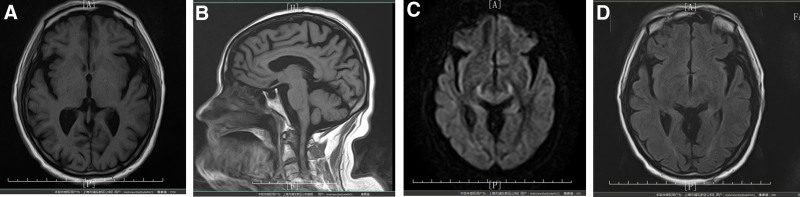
Cranial magnetic resonance imaging (MRI) showed mild atrophy in temporal lobe, cerebellar, and limbic area. T1 sequence (A and B), DWI sequence (C), and T2 FLAIR sequence (D).
